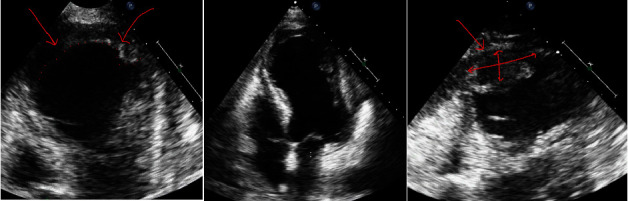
Transthoracic 2D echocardiogram showing severely decreased left ventricular systolic function with global hypokinesis and apical dyskinesis. Large mural layered thrombus in the left ventricular apex. Small to moderate pericardial effusion with no tamponade physiology. Red arrows demonstrate the site of wall rupture.
